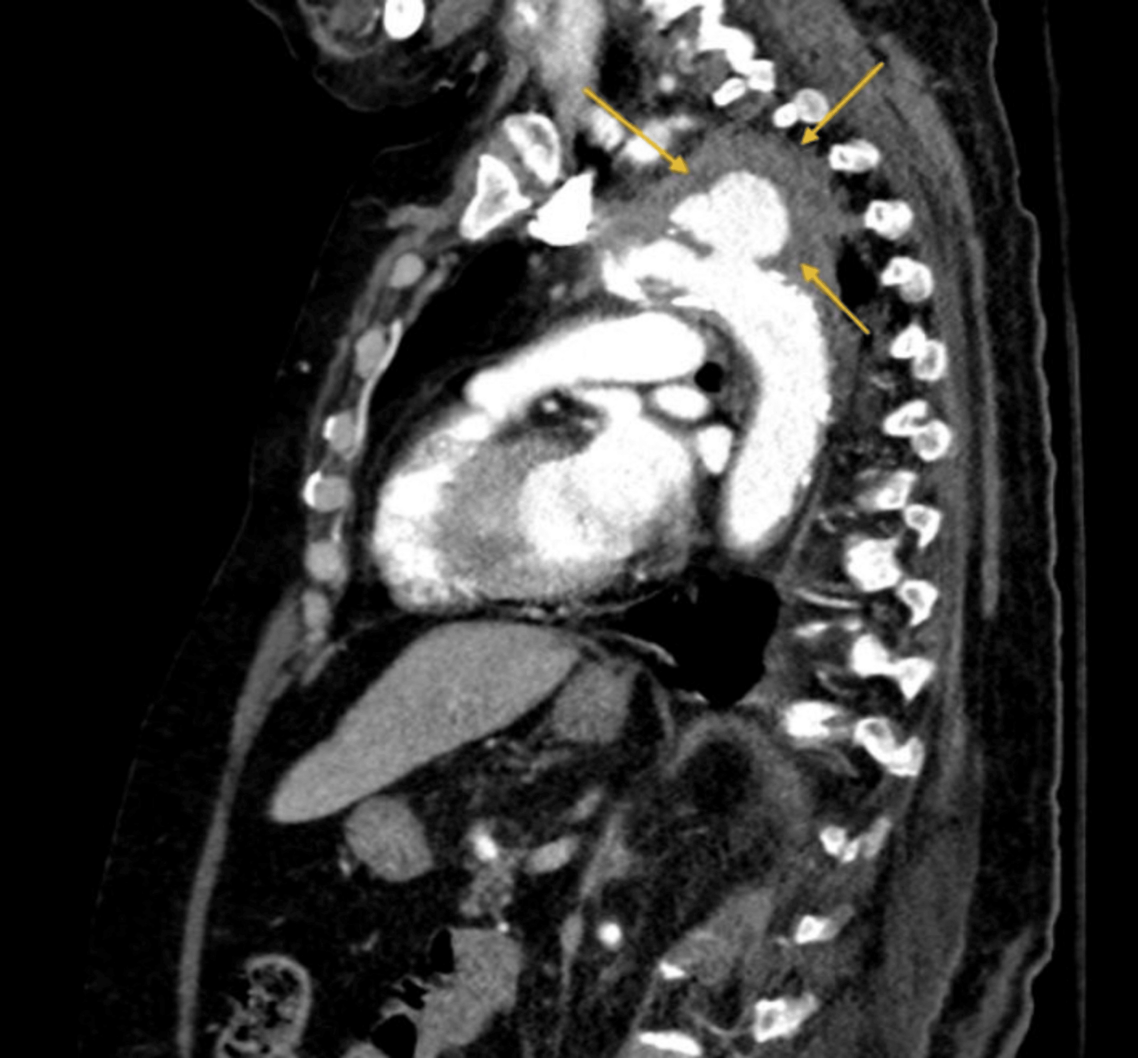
Sagittal view of contrast-enhanced chest CT showing a proximal descending thoracic aorta pseudoaneurysm (yellow arrows) with surrounding hematoma
